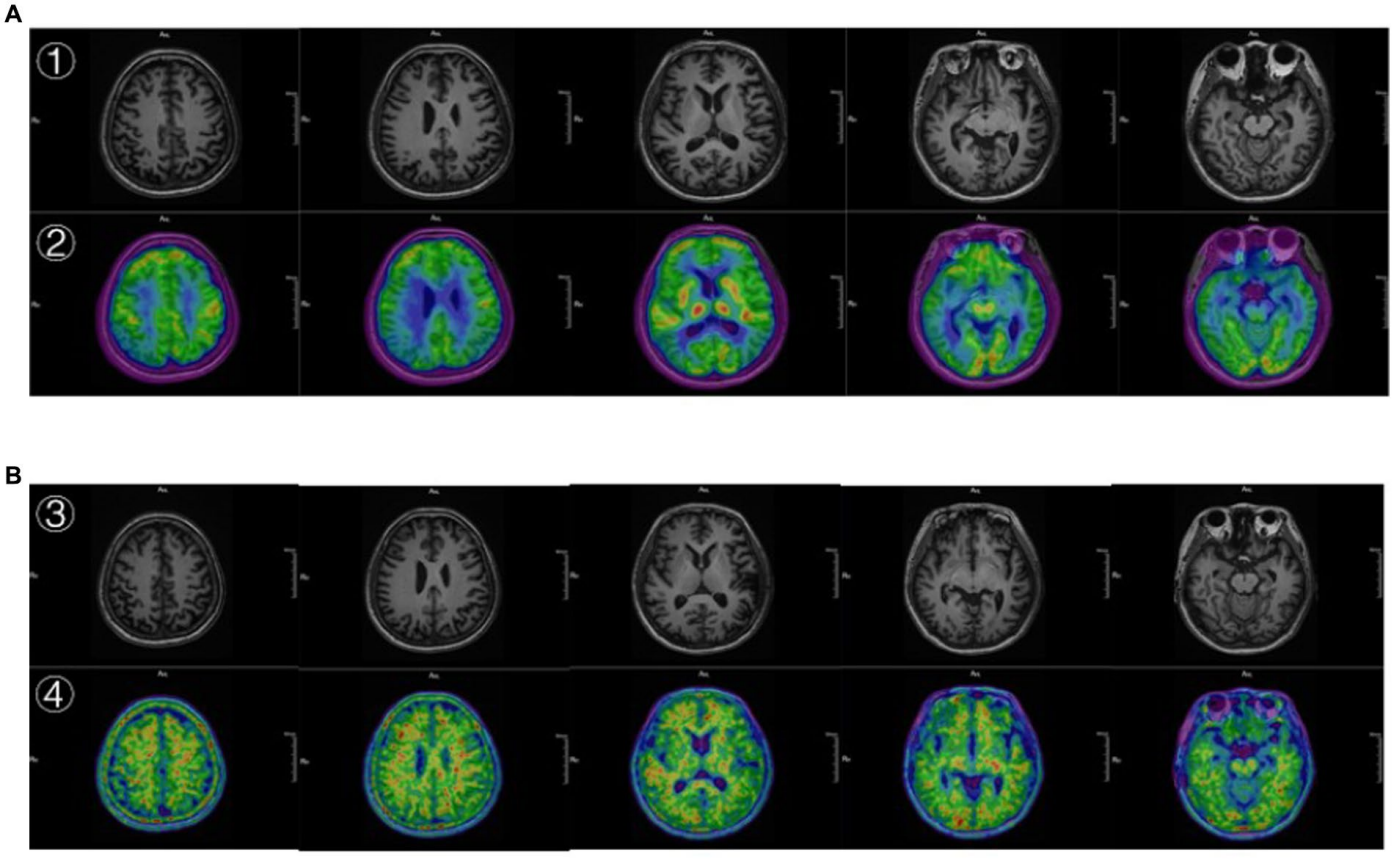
(A) ① The 3D T1 MRI. ② the image ^18^F-FDG PET fused with MRI. ② Showed hypometabolism in the parietal, temporal, and posterior cingulate gyrus. (B) ③ The 3D T1 MRI. ④ The image ^18^F-AV45 PET fused with MRI. ④ Showed significant cortical A*β* deposition.
